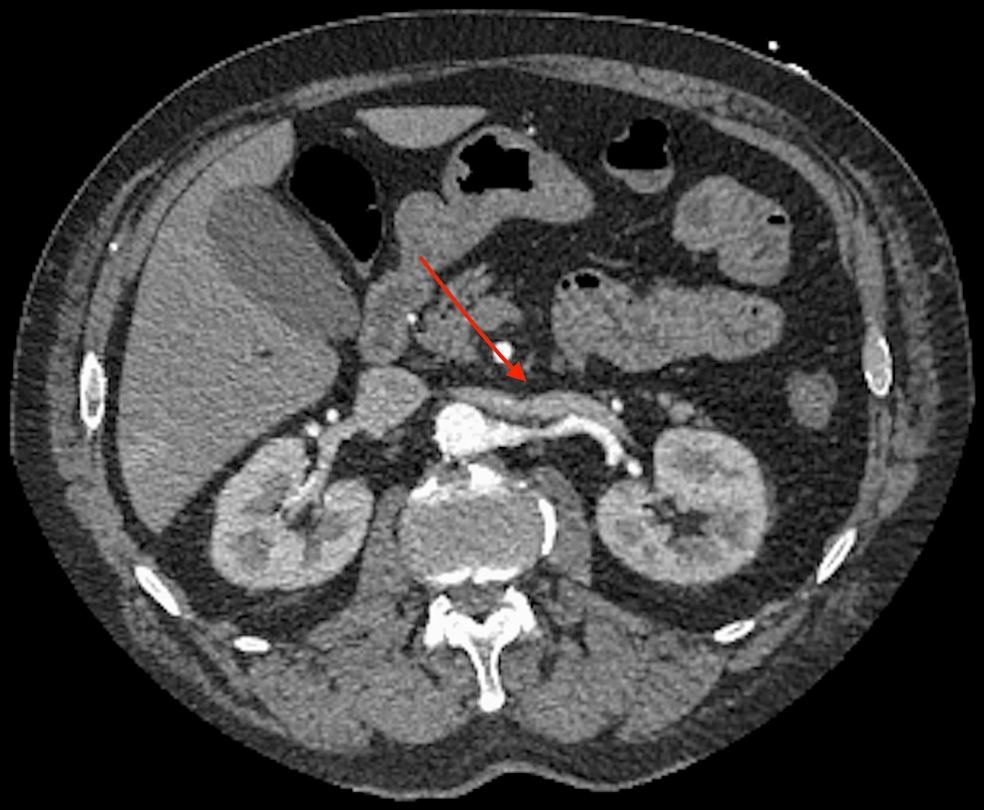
CT scan showing superior mesenteric artery stenosis as indicated by red arrow.
